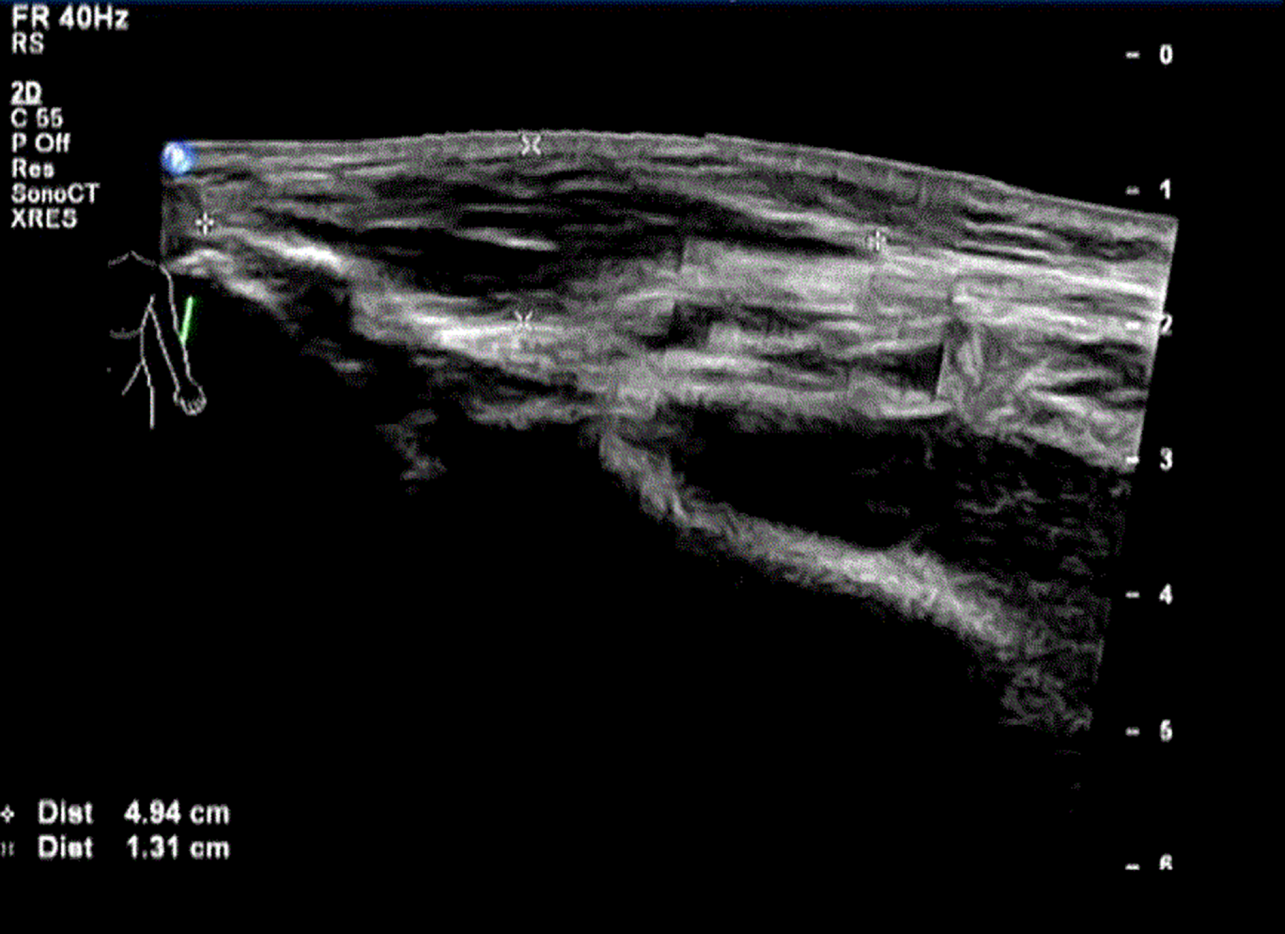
Ultrasonography of left forearm before the diagnosis of focal myositis.
